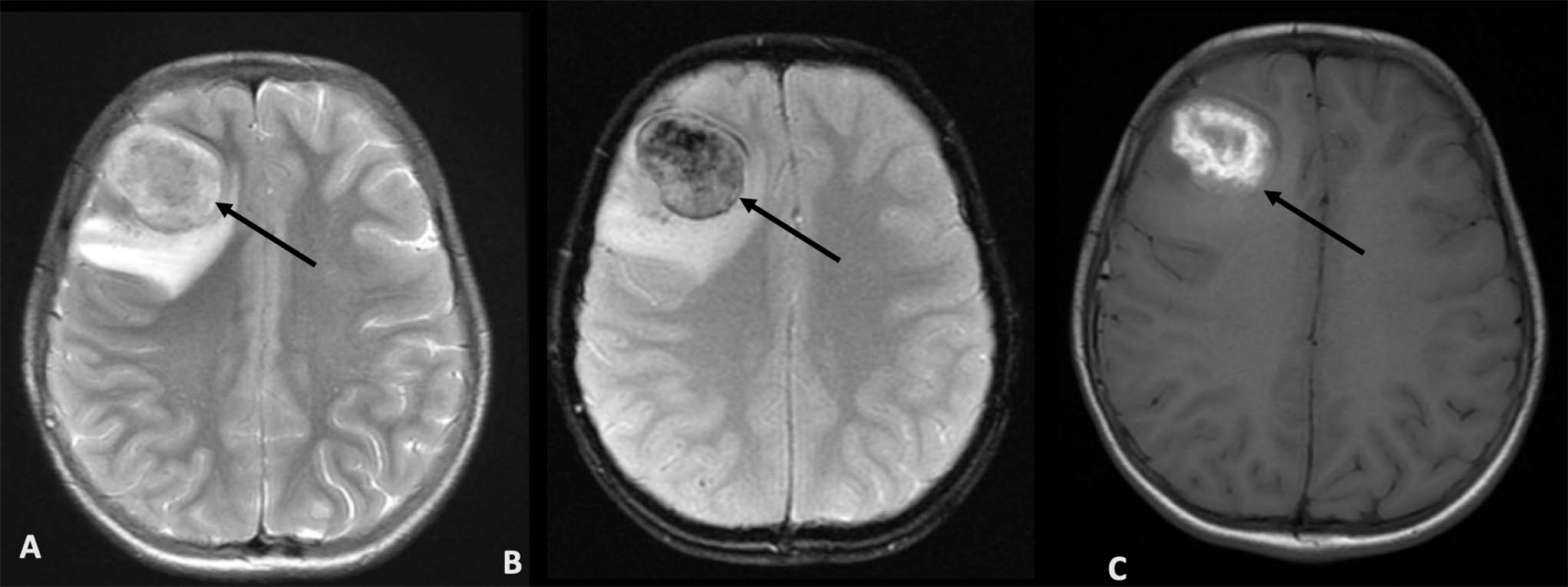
MRI brain images of P9 showing multiple ring-enhancing lesions with surrounding cerebral oedema. Axial T2 weighted images (A), axial T2 gradient (B) and axial pre-contrast T1 weighted images show a round parenchymal mass with internal T1 hyperintense signal (C) and T2 ‘blooming’ (B) which is in keeping with blood products. There is surrounding oedema and mass effect. MRI – magnetic resonance imaging
